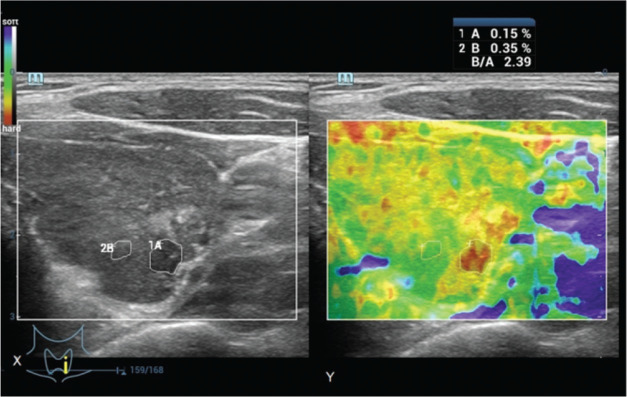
Determination of the strain index of a 42-year-old male patient with abnormal thyroid function test result. The B-to-A ratio was the strain index. X: Real-time ultrasonography. Y: Ultrasound elastography evaluation. B: Thyroid nodule strain. A: The strain of the softest area of the parenchyma. Real-time ultrasonography and ultrasound elastography were performed by ultrasound technologists with a minimum experience of 5 years.
